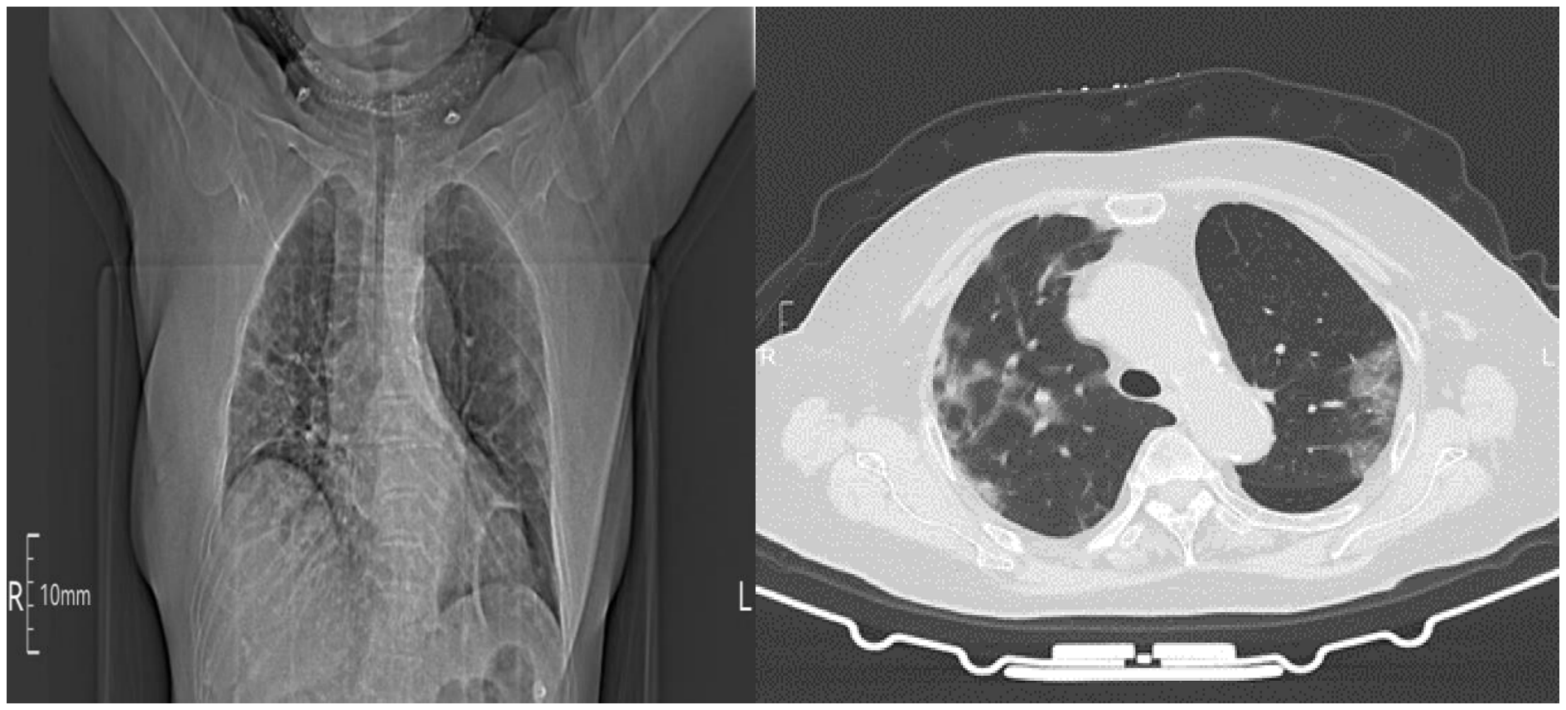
Chest computed tomography (CT) indicated non-regional ground-glass opacities in the lungs and infiltrative shadows.
